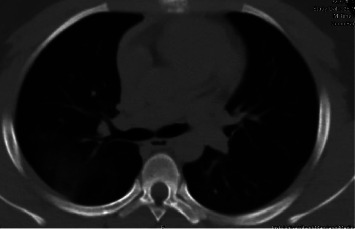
Axial view of CT chest scan showing a significant resolution of bilateral ground-glass opacities after treatment with diuretics.
